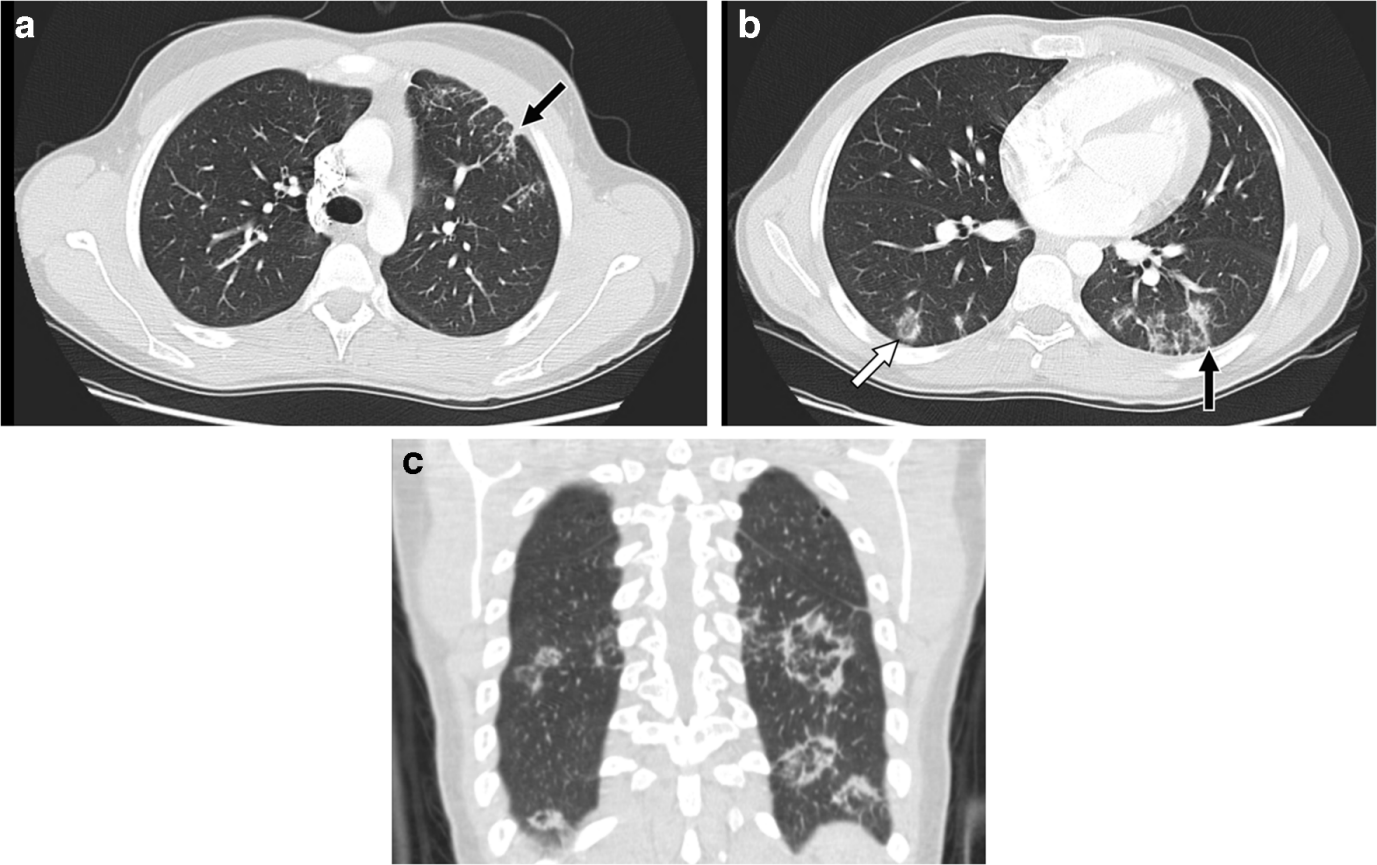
20-year-old male diagnosed with dermatomyositis when he was 17 years old. a, b Axial and (c) coronal CT images with intravenous contrast showing areas of peripheral perilobular opacity (black arrows) and reversed halo sign (white arrow) in the lower lobes and anterior left upper lobe suggesting organizing pneumonia
